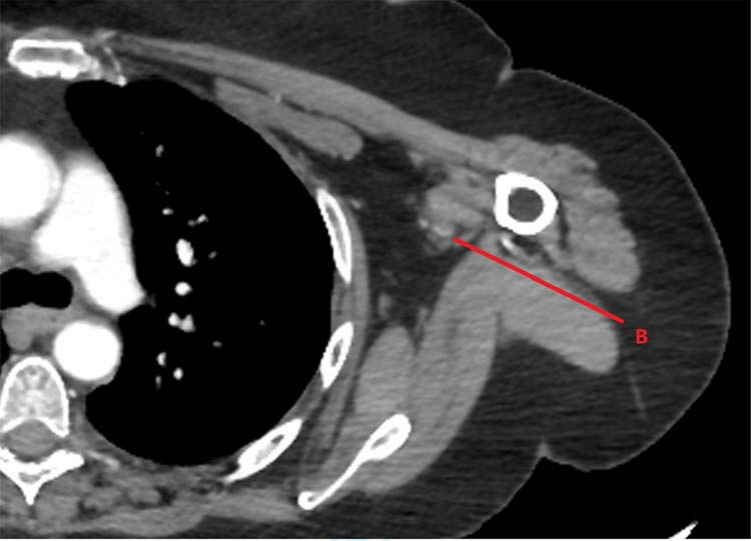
CTA of left upper limb in the transverse plane. B—axillary artery without contrast showing occlusion due to embolism as evident in transverse imaging at B-line (see Fig. 2).
